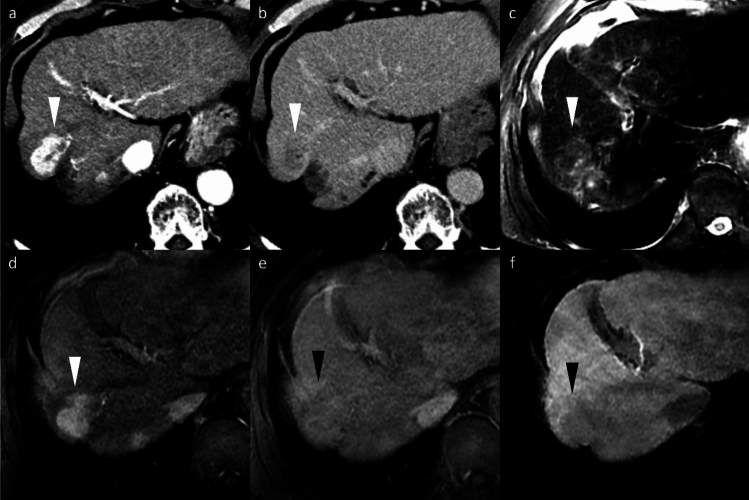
Hepatocellular carcinoma (HCC) in a 71-year-old man with hepatitis C virus-related cirrhosis. HCC (arrowheads) displays non-rim arterial hyperenhancement on the arterial phase CT image (a) and portal venous washout (b; arrowhead). HCC shows inhomogeneous hyperintensity on the fat-suppressed T2-weighted image (c) and demonstrates non-rim arterial hyperenhancement on the arterial phase image (d). In contrast, neither portal venous washout (e) nor hypointensity on the hepatobiliary phase image (f) is clearly observed. Severe liver dysfunction due to advanced cirrhosis decreases gadoxetic acid uptake, thereby reducing the diagnostic contrast between HCC and background liver parenchyma
